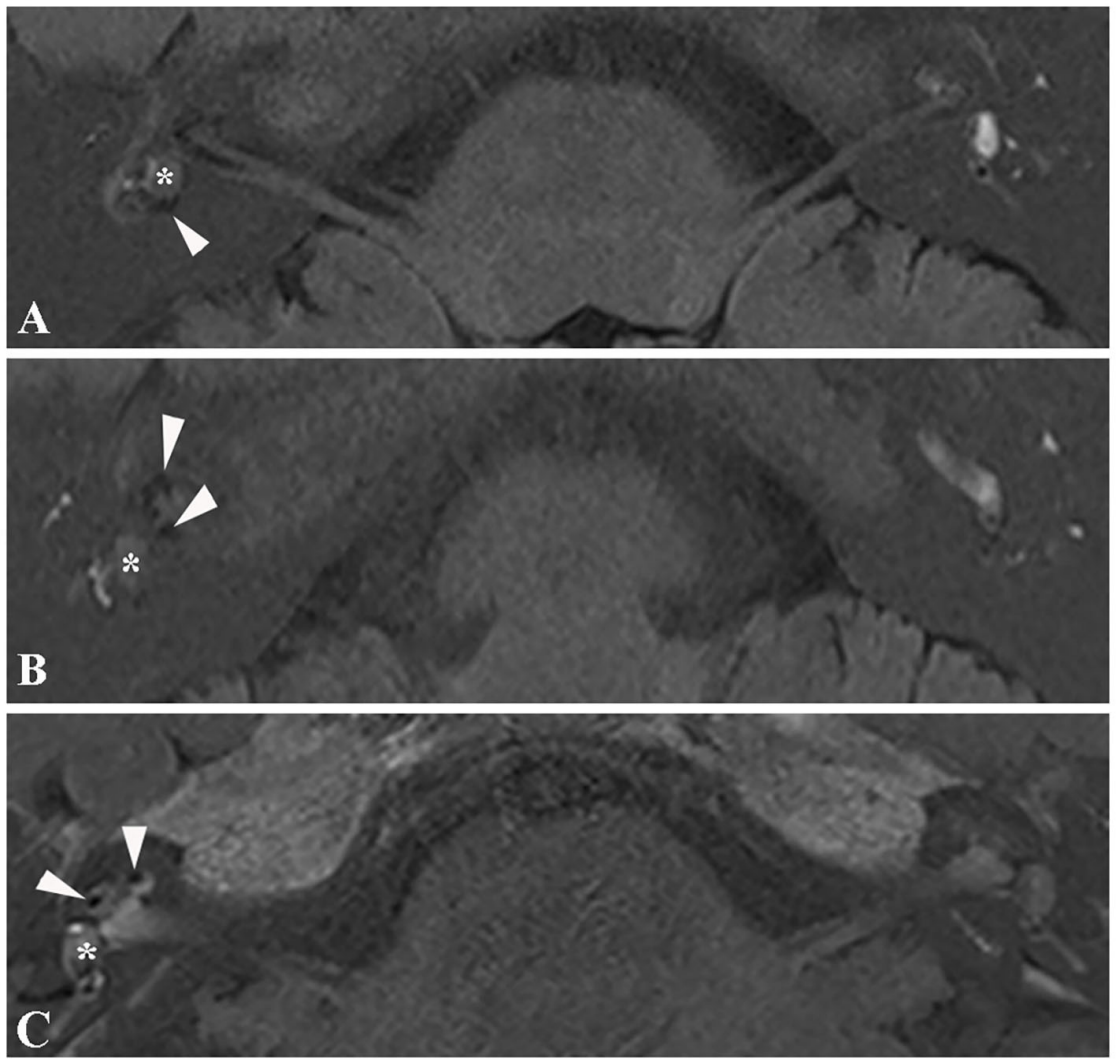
Magnetic resonance image of endolymphatic hydrops. (A) 3D real IR MRI of patient 6 shows endolymphatic hydrops in the vestibule (arrowhead) concurrent with an intravestibular tumor (asterisk). (B) 3D-real IR MR image of patient 6 with an intravestibular tumor (asterisk), presenting with endolymphatic hydrops in the cochlea (arrowhead). (C) 3D-real IR MRI of patient 4 with intravestibular tumor (asterisk) showing endolymphatic hydrops in the basal turn of the cochlea.
